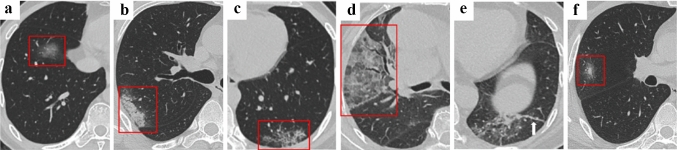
CT images of most common COVID-19 CT findings. Adapted from [6]. a Ground-glass opacity. b Consolidation. c Reticular pattern. d Crazy-paving pattern. e Fibrosis. f Halo sign
